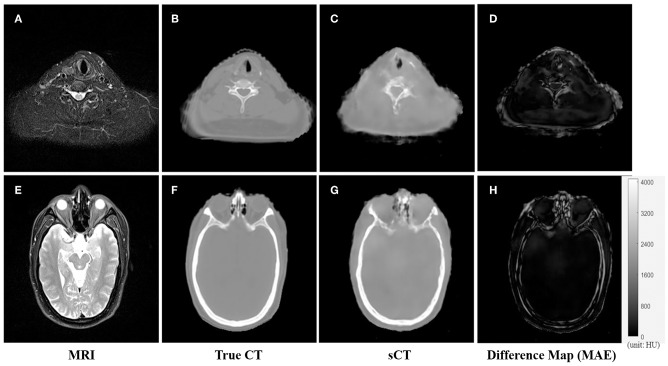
The comparison of CT and synthetic CT for two representative MR images. (A) MR image in the neck region; (B) real CT image aligned with (A); (C) synthetic sCT converted from MR image (A); (D) difference map between (B,C); (E) MR image in the head region; (F) real CT image; (G) synthetic sCT image; (H) difference map between (F,G). Gray bar in (H) indicated the mapping from CT number to gray scale in the difference maps.
